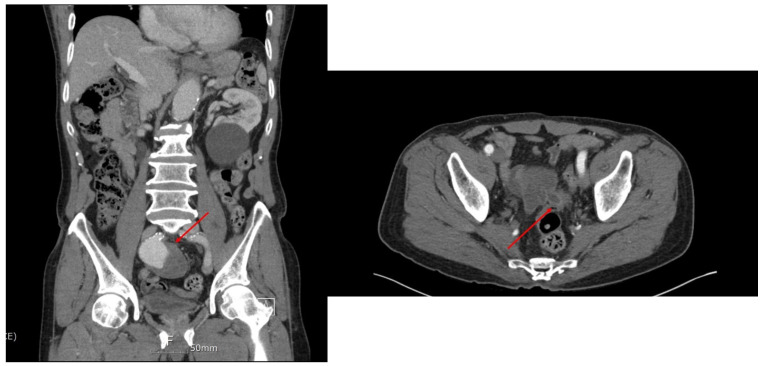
Pre-operative computed tomography showing internal iliac artery aneurysm and fistula tract in sigmoid colon.
